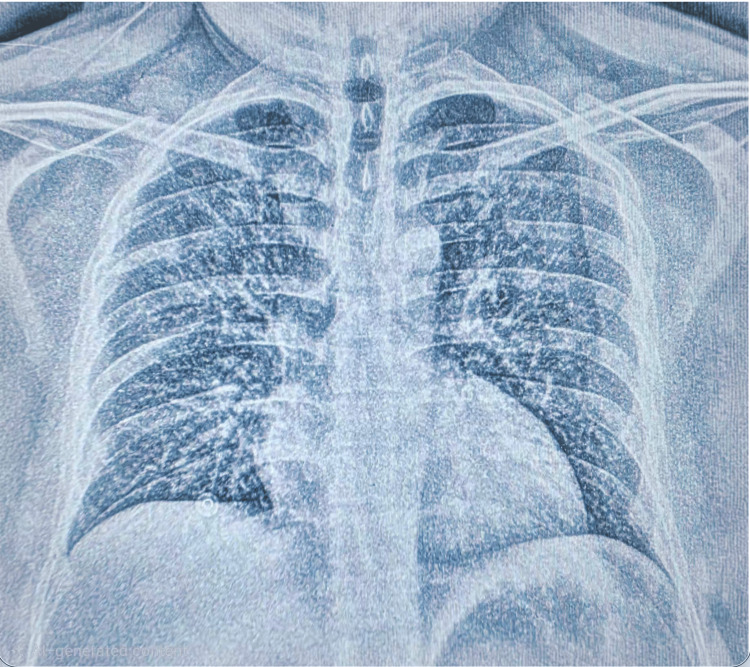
Anteroposterior chest X-ray: portable chest X-ray showing hypermarkings of vascular airways
